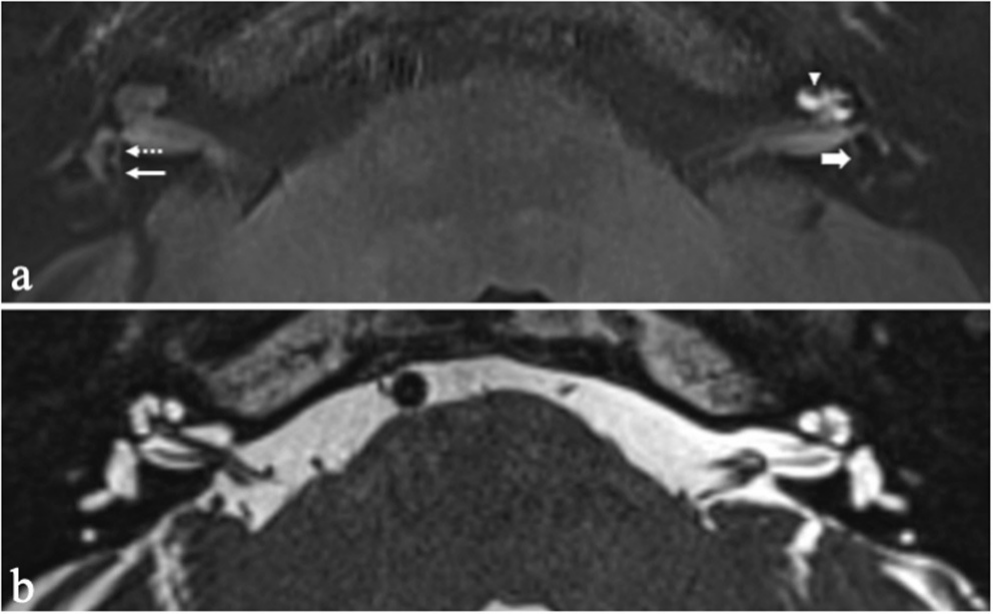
Axial 4 h-delayed Gd-enhanced 3D FLAIR MRI (a) and axial heavily T2 weighted sequence (b) in a patient with recurrent vertigo attacks and left-sided sensorineural hearing loss. The affected left ear demonstrates dilation of the scala media with complete obliteration of the scala vestibuli compatible with grade 2 cochlear hydrops (arrow head). The saccule and utricle in the left ear are confluent indicative of grade 2 vestibular hydrops (thick arrow). A thin rim of surrounding perilymph remains visible. Compare with a normal saccule (dashed arrow) and utricle (thin arrow) in the right ear. Also note the increased perilymphatic enhancement in the cochlea of the left ear, suggestive of BLB impairment.
